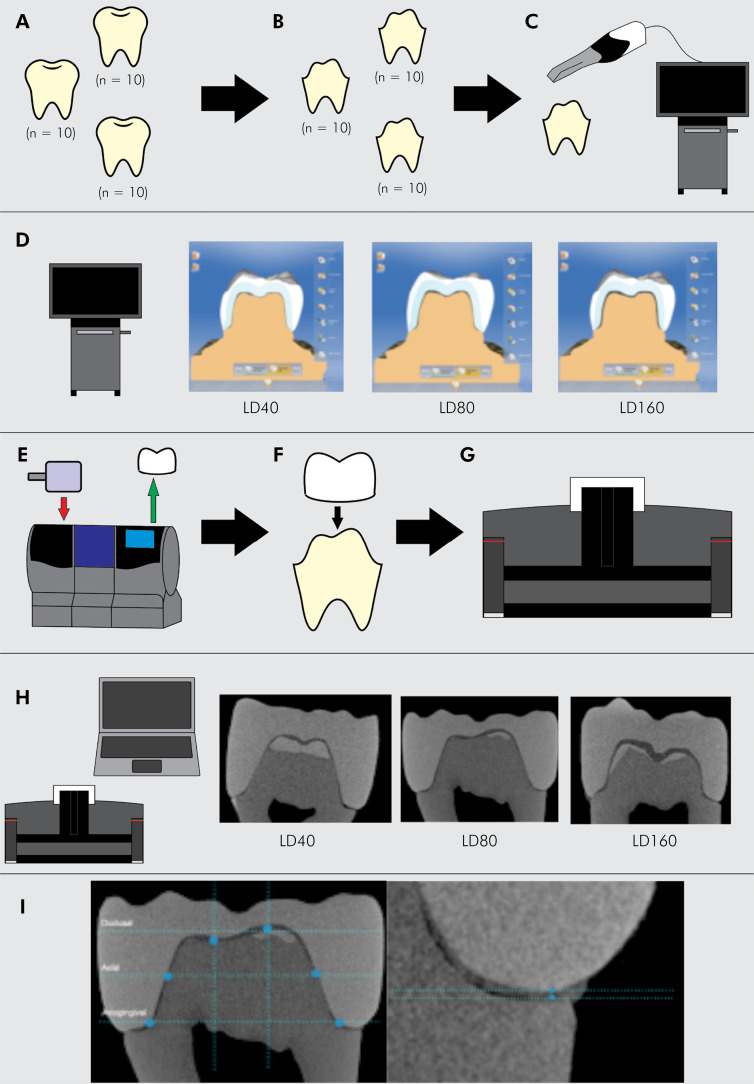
Experimental methodology. (A) Tooth selection, (B) tooth preparation, (C) scanning process, (D) crown design, (E) crown fabrication, (F) fixation process, (G) micro-computed tomography, (H) reconstruction, and (I) internal and marginal measurement procedures.
